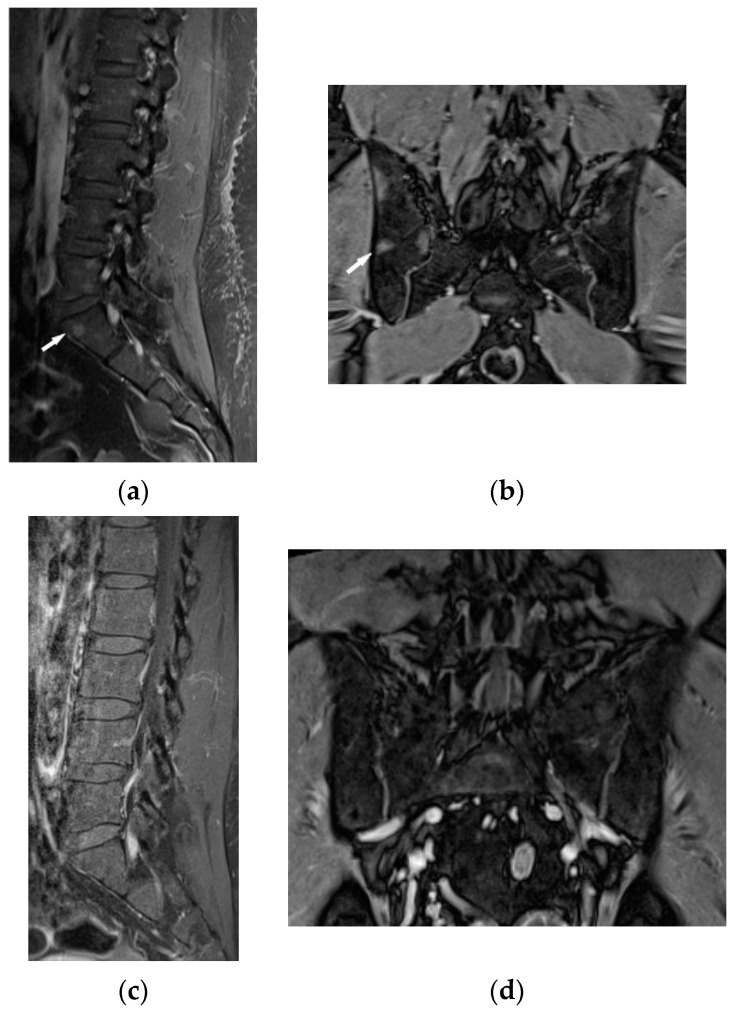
34-year-old patient. T1-weighted images, contrast enhanced, with fat saturation of the lumbar spine and pelvis. Multiple enhanced bone marrow lesions in the vertebral bodies ((a), white arrow) and in the pelvis at the level of the sacroiliac joints ((b), white arrow). Complete regression of the lesions after the treatment in the vertebral bodies (c) and pelvis (d).
